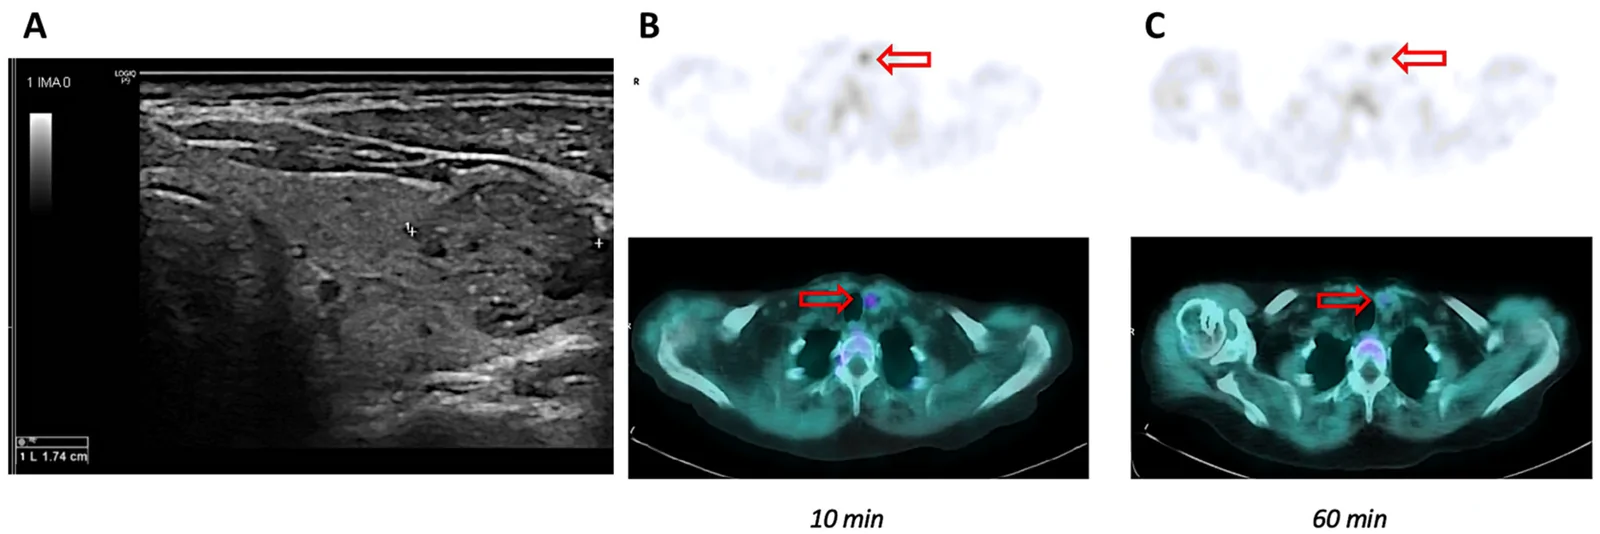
Neck US and [^18^F]FCH of a patient with pHPT (PTH 83.1 ng/L, Calcium 9.3 mg/dL). (A) Neck US showed a thyroid nodule in the left lobe but did not detect hyperplastic parathyroid tissue; (B) Early-phase images (PET and fused PET/CT) obtained 10 min p.i., showing a focal [^18^F]FCH uptake corresponding to a small nodular lesion located in the left inferior lobe of the thyroid gland (LC = 52.1%). (C) Late-phase images (PET and fused PET/CT) obtained 60 min p.i., showing a decreased uptake of the same lesion (RI = −32.87%; LC = 2.1%), indicating a rapid washout of the hyperfunctioning parathyroid lesion (arrow).
